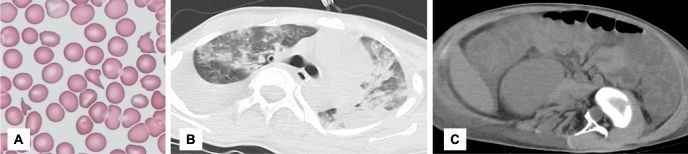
(a) Fragmented red blood cells are found in peripheral blood smears. (b) Chest CT showing diffuse bilateral ground-glass opacity, consolidation, and interlobular septal thickening. (c) Abdomen CT showing intestinal wall thickening and ascites
